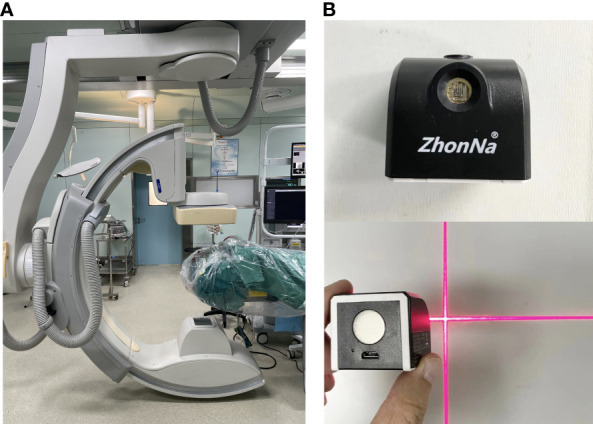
(A) Xper CT equipment. (B) Laser locator.
